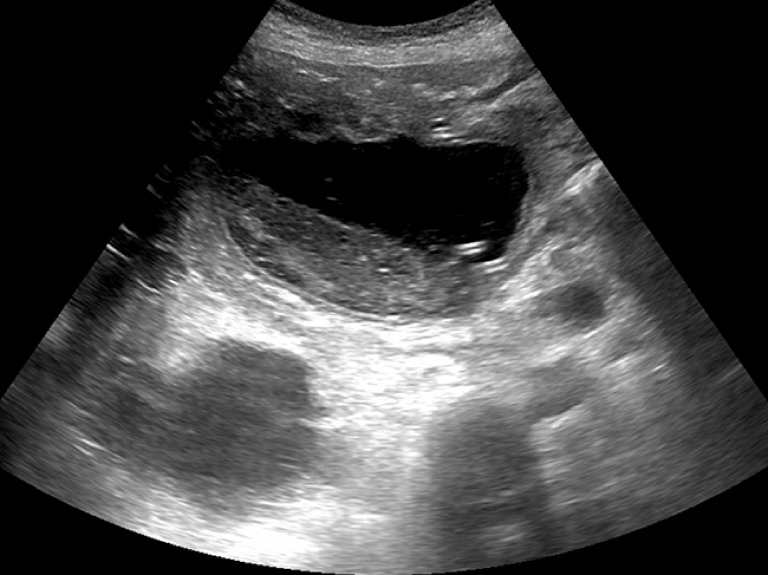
Sonography 1 month later showed a decrease in cyst size, with residual debris inside.
